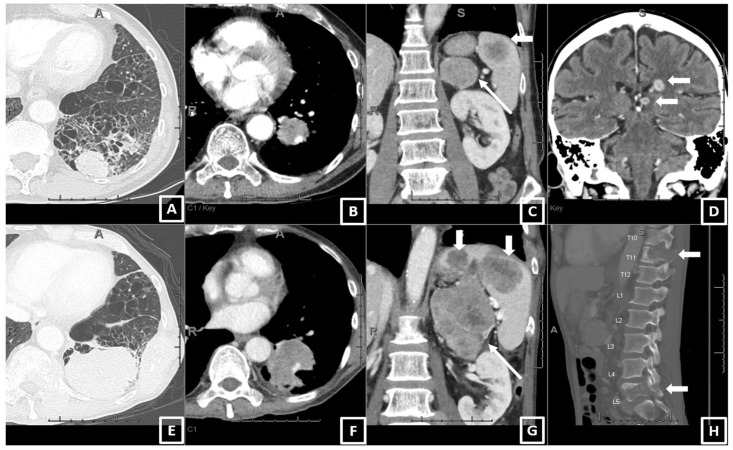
An example of a patient who had PD with pembrolizumab monotherapy and had a high value for the predicted risk of progression (0.93) using the prediction equation and the Youden cut-off. A 64-year-old male, current smoker, with 30 pack years, ECOG 2, diagnosed as stage IV NSCLC (adeno.). The baseline CT (A–D) showed (A) a 3.5 cm left lower lobe (LLL) primary lung mass, (B) a 2.2 cm LLL lobar adenopathy (also at the hilum and mediastinum, now shown), metastasis in the left adrenal and spleen (long and short arrows in (C)), and brain (D). After 3 months on pembrolizumab, the patient developed progressive disease on the 1st FU CT (E–H), including enlarging the LLL mass (6.1 cm, (E)), lobar adenopathy (3.2 cm, (F)), enlarging and new metastasis in the left adrenal and spleen (G), and worsening destructive bone metastases (H). The brain metastasis was radiated and improved. The patient died 7 weeks after the FU CT.
